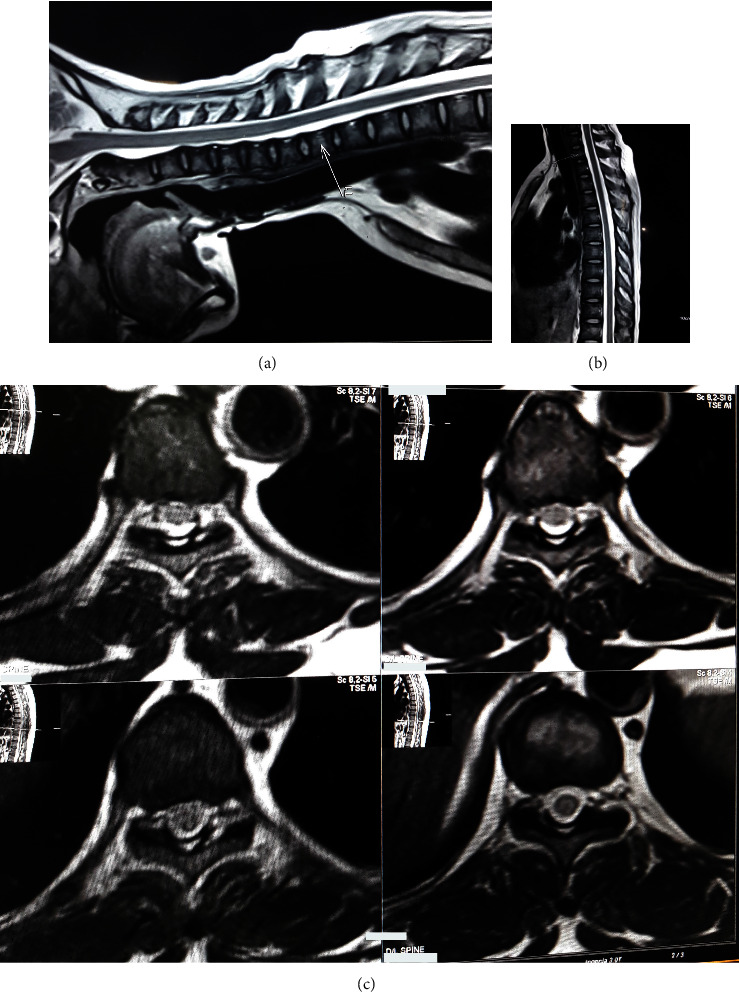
MRI cervical and thoracic spine: (a, b) T2 sagittal and (c) axial view, showing no abnormality.
